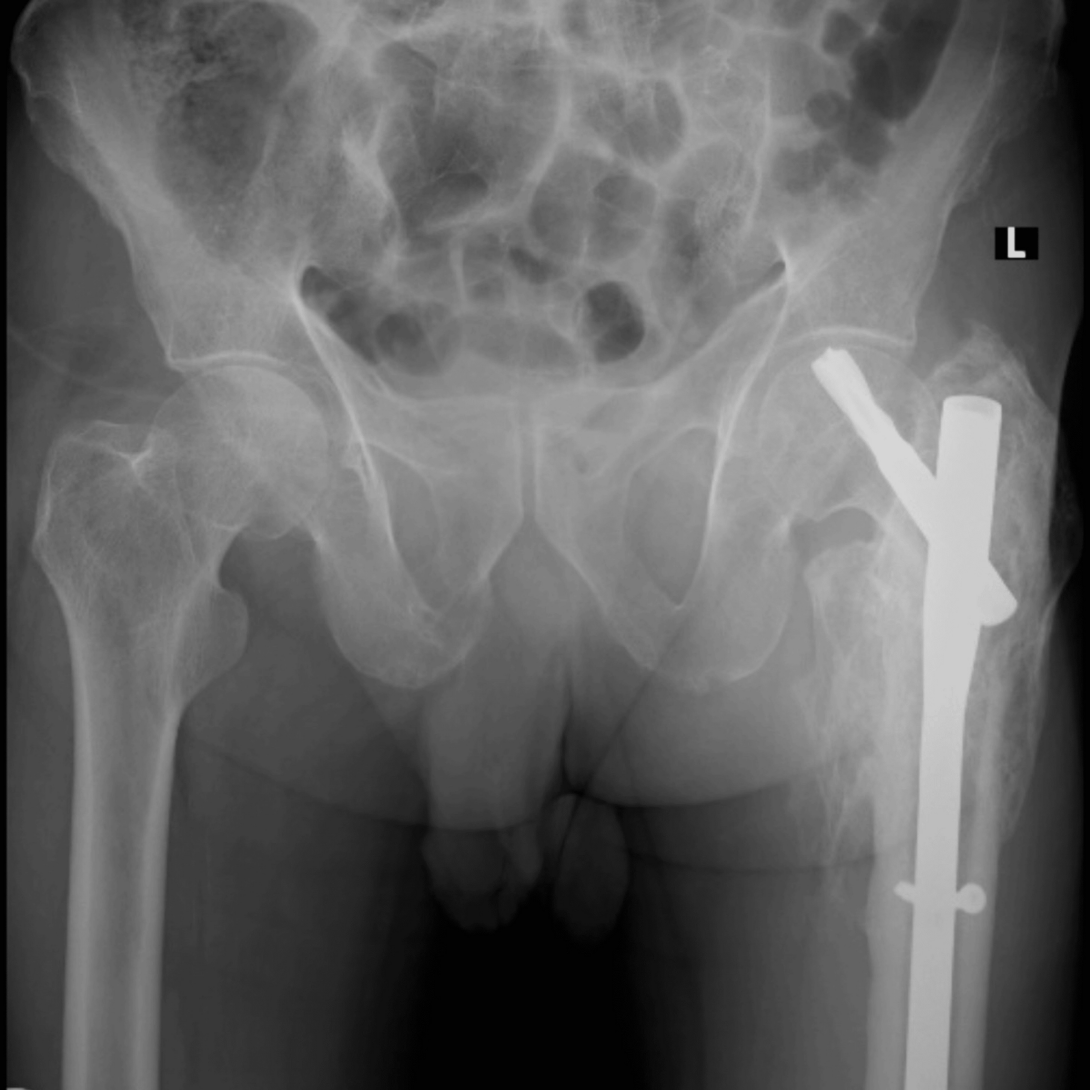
Pelvic radiograph (AP view) showing bilateral sacroiliac joint ankylosis with evidence of left-sided hip arthroplasty. The findings are typical of advanced ankylosing spondylitis with involvement of axial and appendicular skeleton AP: anteroposterior
